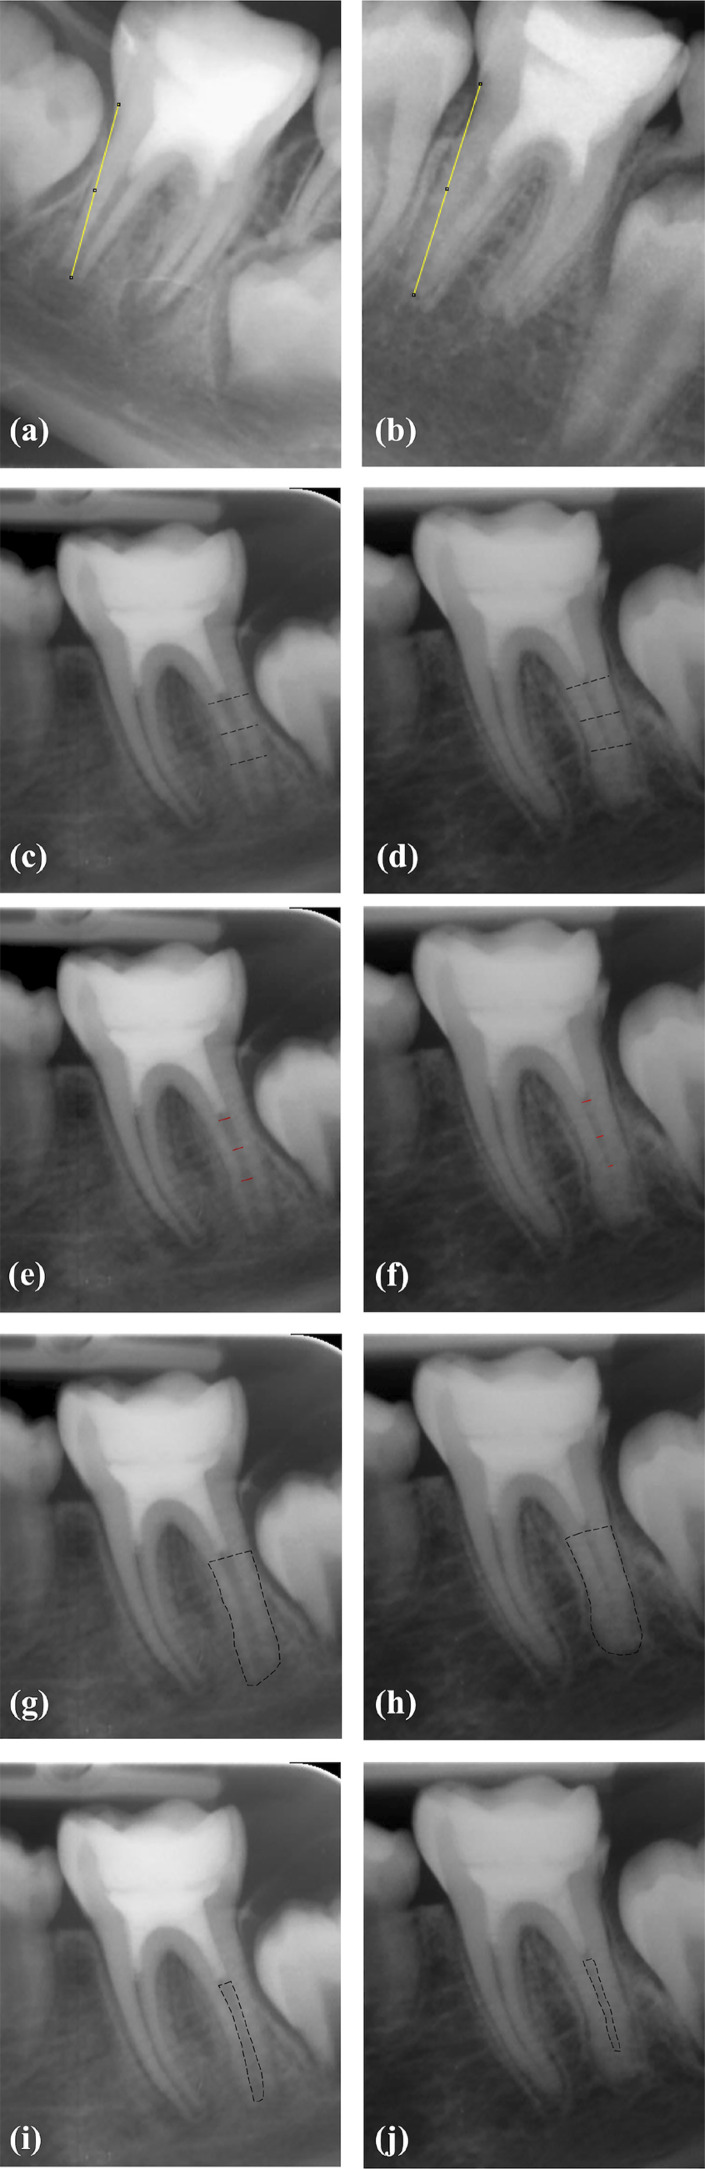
Linear measurements on mathematically aligned radiographic images. Root length: (a) post-operative, (b) 12th month. Root width (c, e) post-operative and (d, f) 12th month (The average root width for preoperative and 12th month images was calculated as; cervical root width-cervical pulp width) + (middle root width -middle pulp width) + (apical root thickness -apical pulp width)/3). RRA (g, i) post-operative and (h, j) 12th month (RRA was defined as the difference between the total root area and the root canal space).
